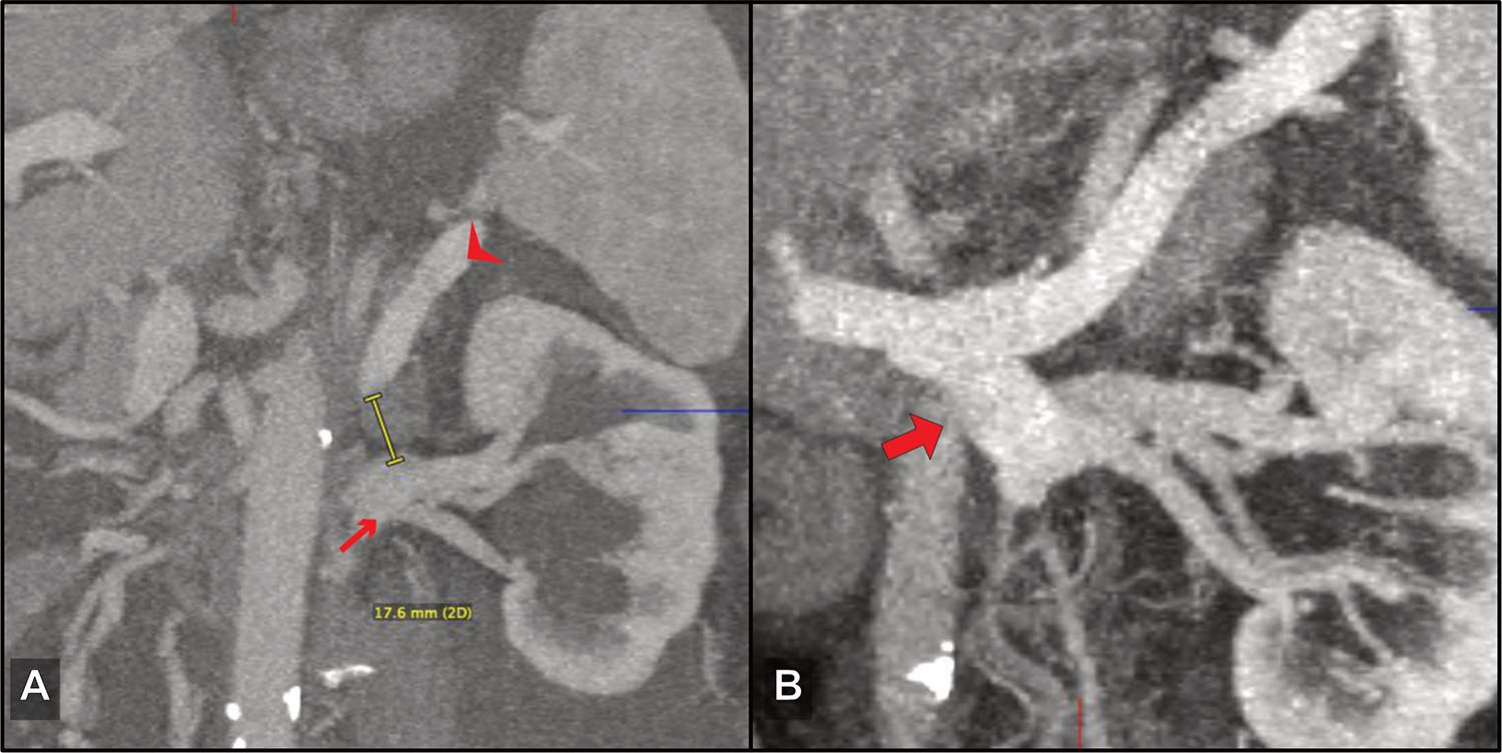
A 71-year-old male PHT patient with excessive ascites after extended right hemihepatectomy (diagnosis: intrahepatic cholangiocarcinoma)—PSSS: splenorenal side-to-side; a preoperative CT, oblique MIP reconstruction and b postoperative CT, oblique MIP reconstruction. Small arrow: left renal vein; arrowhead: splenic vein; bold arrow: splenorenal anastomosis
